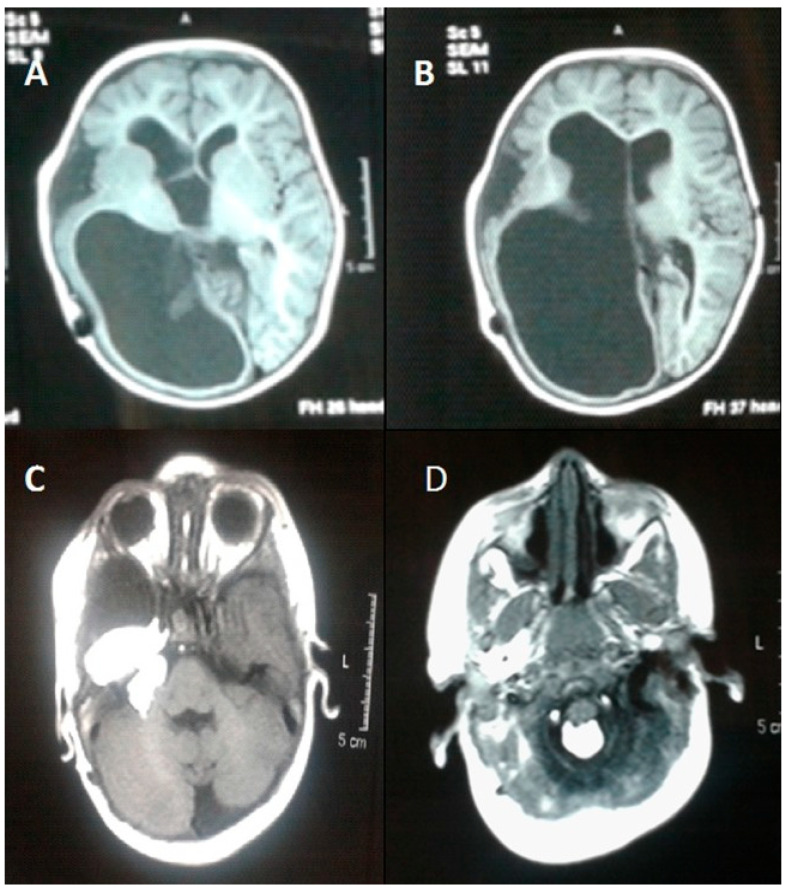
A representation of CNS features associated with ECCL via T-1 weighted magnetic resonance imaging of the brain. (A) Intravenous contrast showing, (B) dilation of the lateral ventricle and atrophy of the right cerebral hemisphere with prominence of the overlying subarachnoid spaces, (C) a fat containing mass measured at 25 × 12 mm visible at the right cerebellopontine cistern angle, and (D) similar fatty lesions in the posterior aspect of the magnum foramen and cervical canal. Reproduced from Naous et al. (2015) [39] with permission as per the CC BY-NC 3.0 license.
